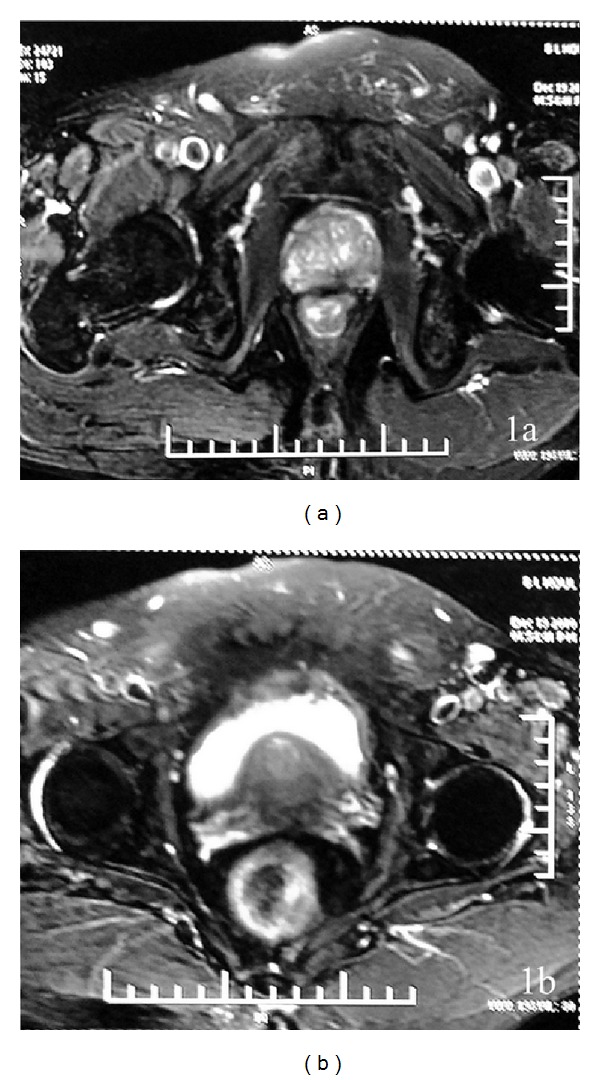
MRI showed ill-defined low intensity signal in peripheral zone of prostate and adjacent portion of bilateral seminal vesicles.
